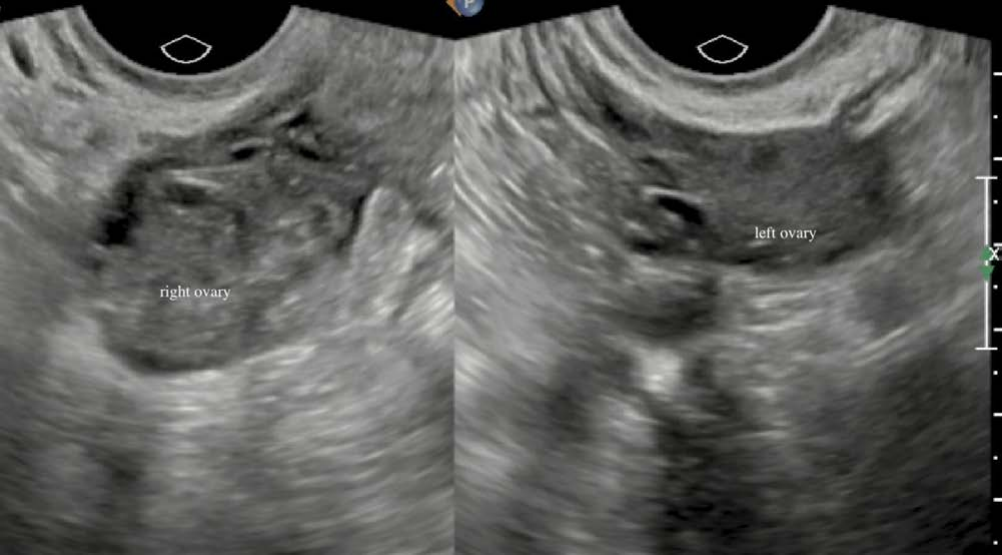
Two-dimensional transvaginal ultrasound image obtained 1 month after surgery showing regression of cysts in the right ovary. Note that both ovaries were normal in size.
